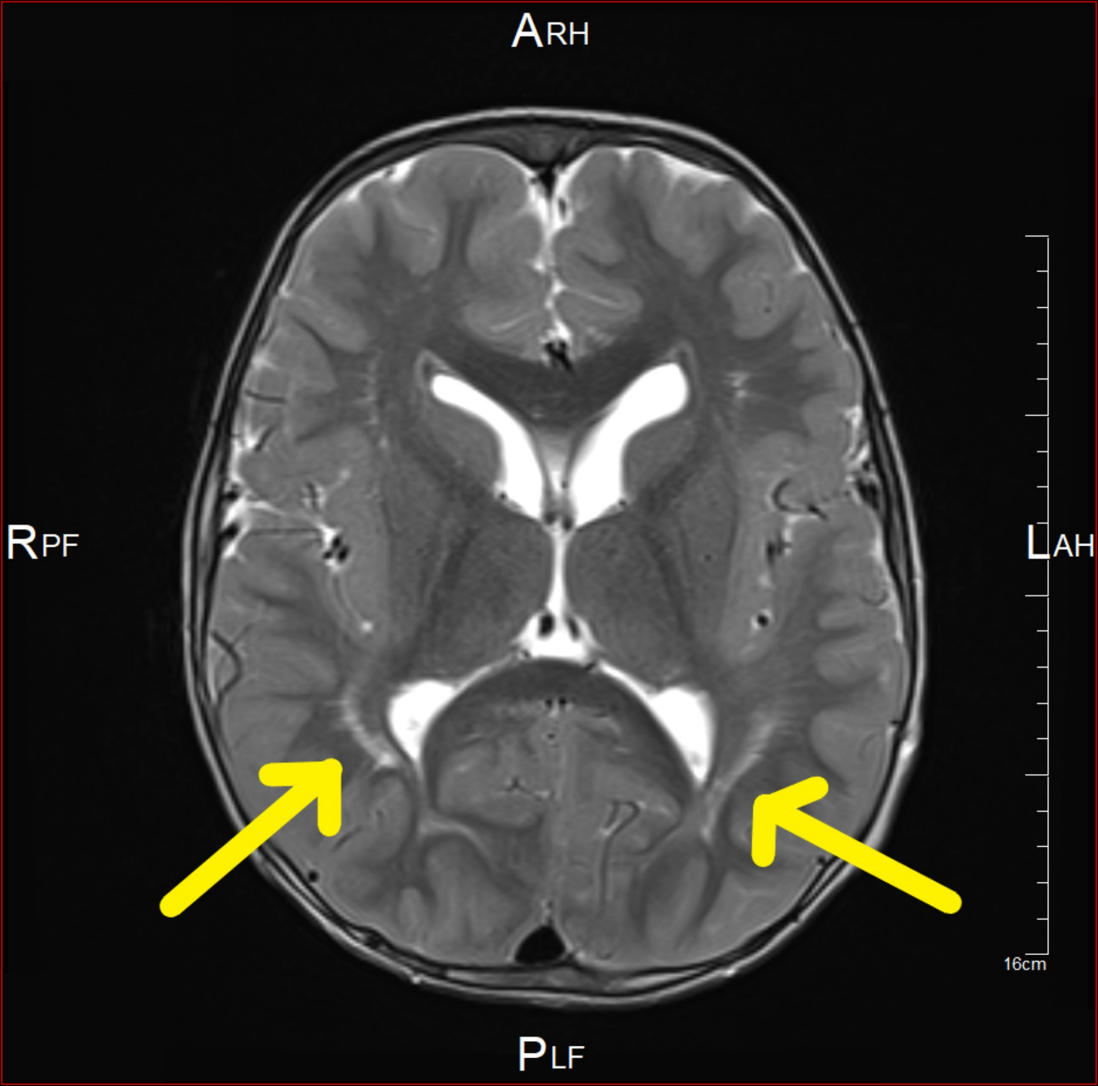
Brain MRI showing bilateral peri-regional high T2 signal intensity
